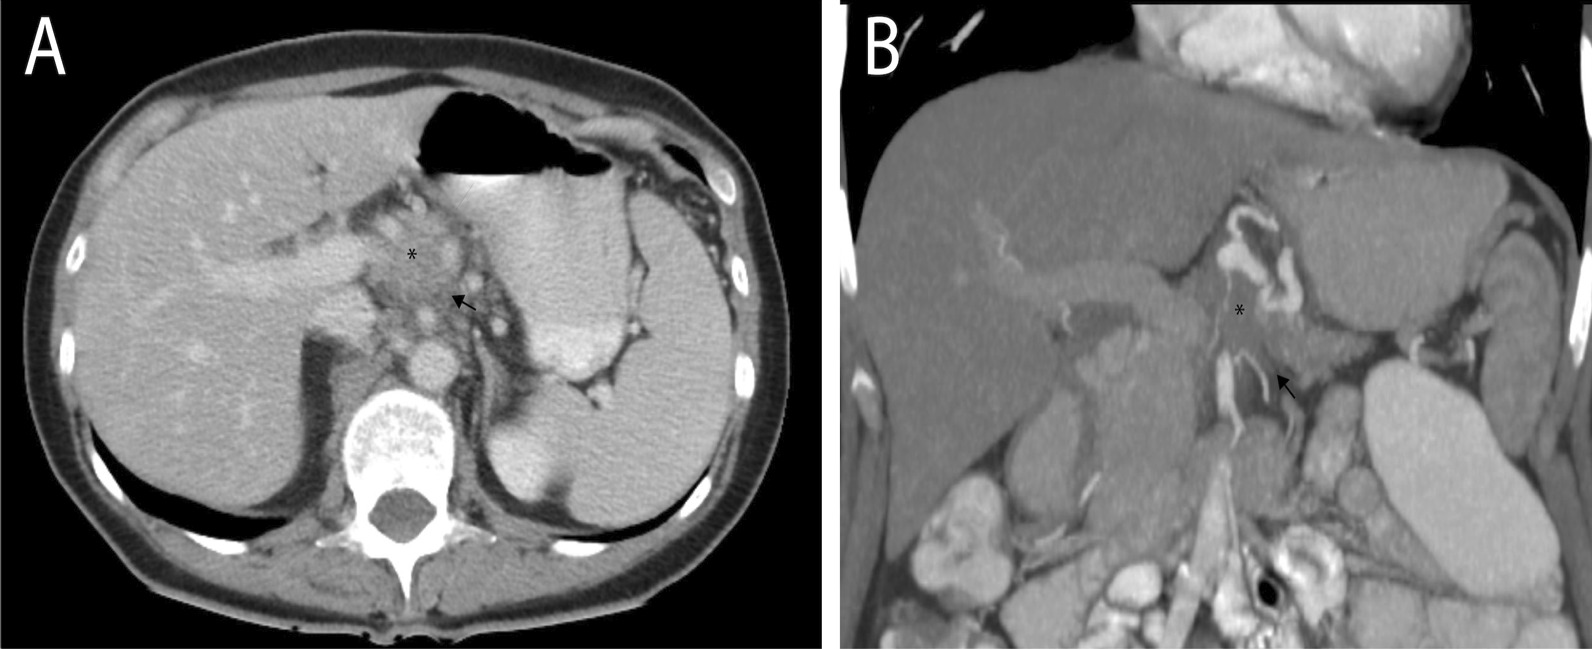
A Axial reconstruction of computed tomography (CT) scan showing the tumor of the pancreas (*) with infiltration of peripancreatic fat tissues (black arrow) B Coronal reconstruction
